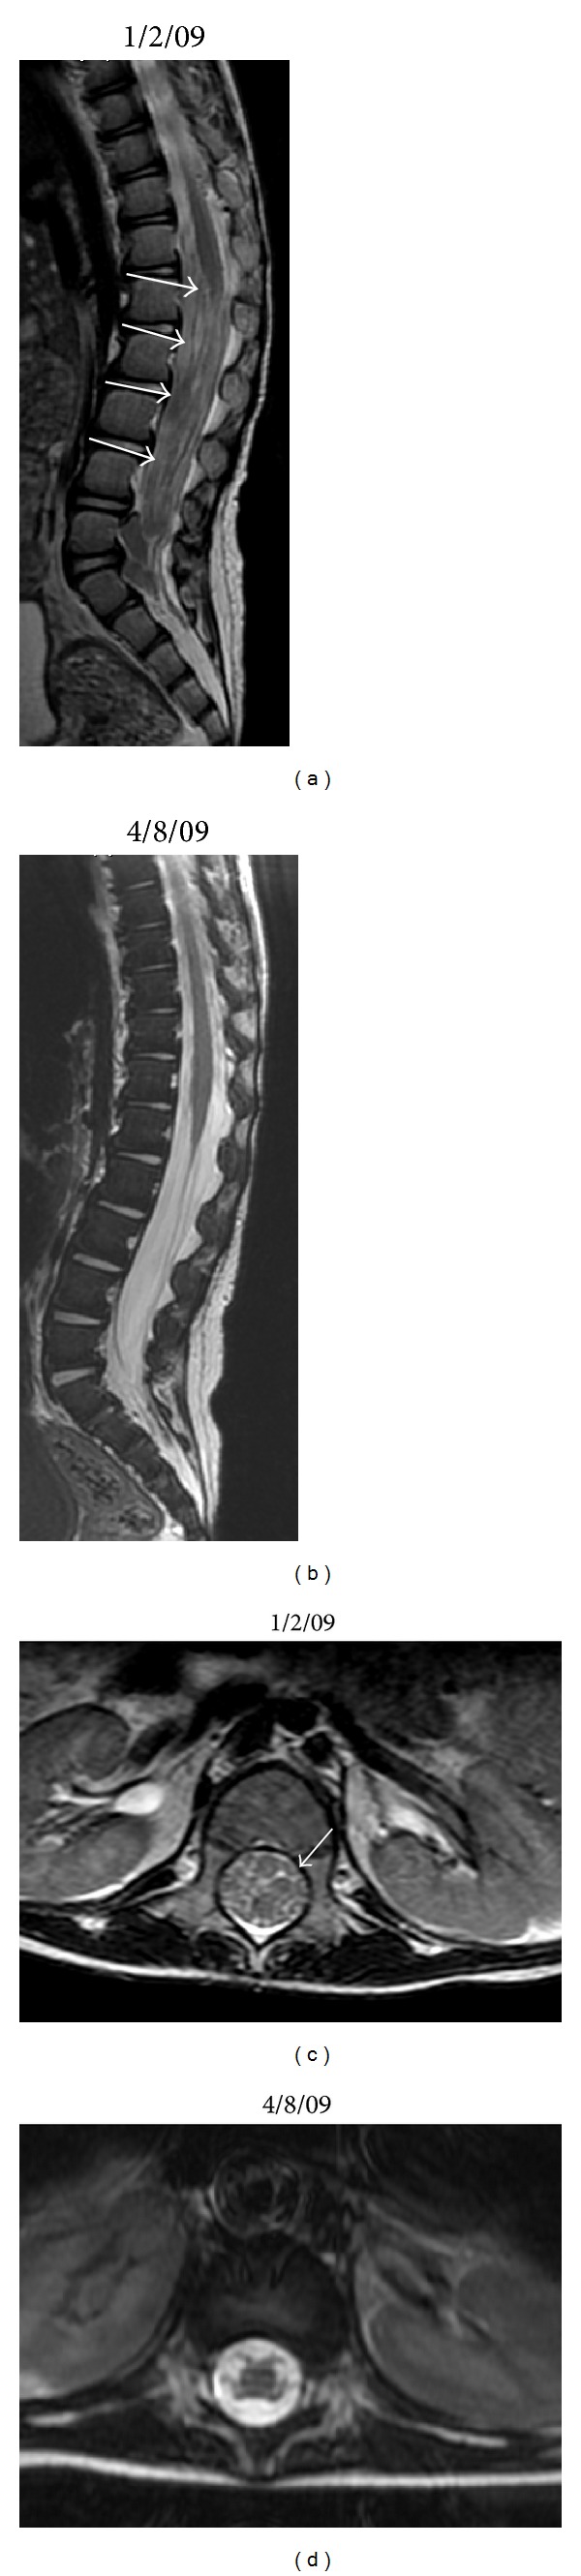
(a) 1/2/09 MRI spine T2 weighted image shows extensive abnormal and nodular enhancement filling the thecal sac from T11-12 extending down through the lumbosacral spine (arrows). (b) 4/8/09 MRI spine T2 weighted image shows near resolution of previously seen abnormal enhancement. (c) 1/2/09 MRI spine axial image demonstrates enhancing masses and thickened nerve roots filling the thecal sac at L1. (d) 4/8/09 MRI spine axial image with normal appearance of thecal sac and nerve roots at L1.
